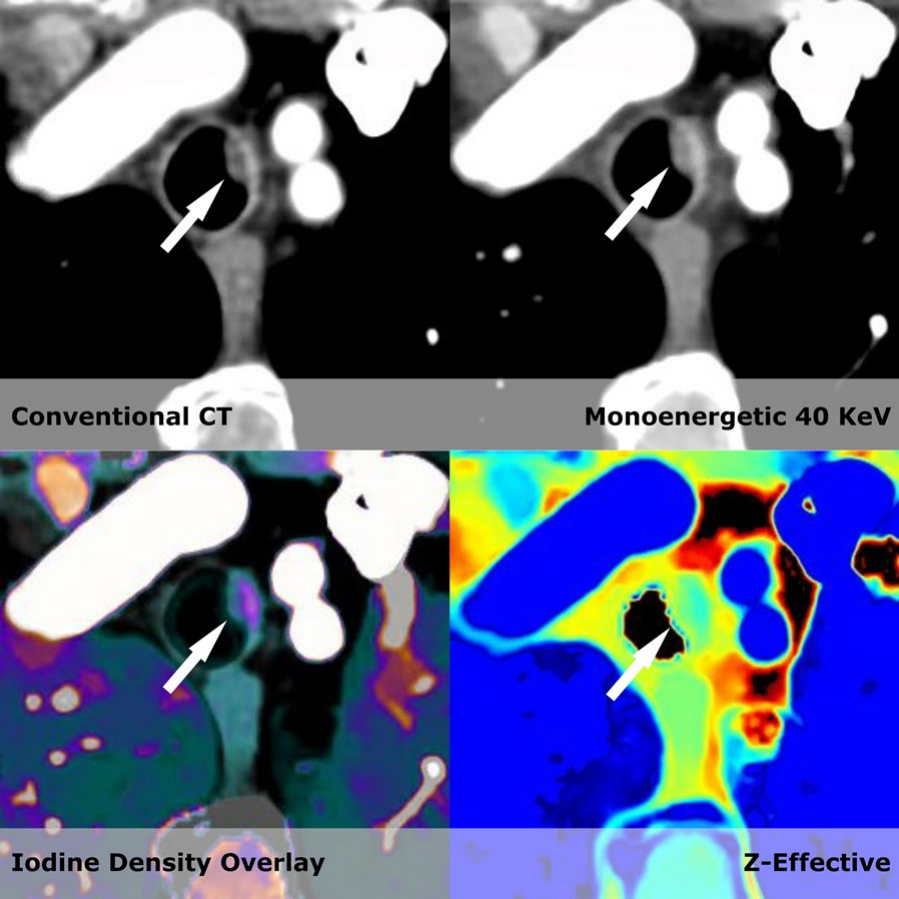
On the left side of the trachea a lesion traversing the wall with a soft-tissue component are seen (white arrow). However, the lesion could also represent intratracheal mucus. But during the reading of the CE-CT, it was missed. On the CE-SCT it was seen both on the virtual low monoenergetic and the Z-effective map which led to a suggestion of flexible bronchoscopy. Unfortunately, the patient was deceased by the time the CE-SCT was read and no definitive diagnoses were made
